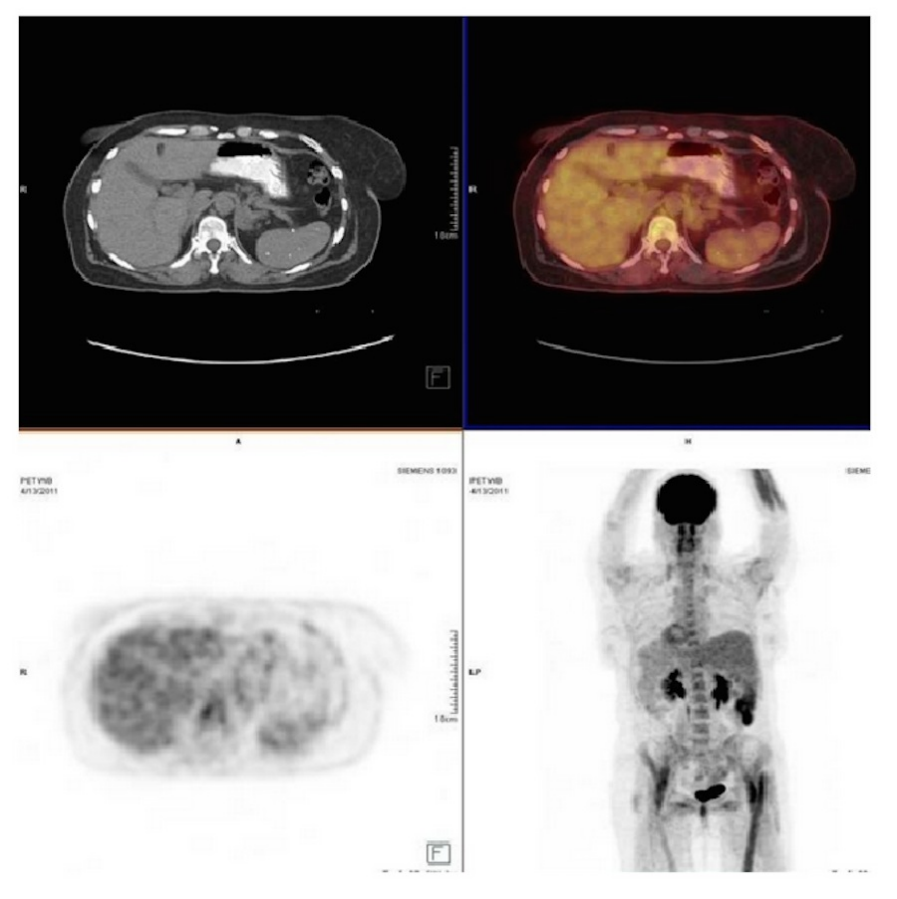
Post-therapy FDG-PET/CT No evidence of FDG-avid nodal lesions suggestive of resolution of previously noted FDG-avid lymphomatous disease.
